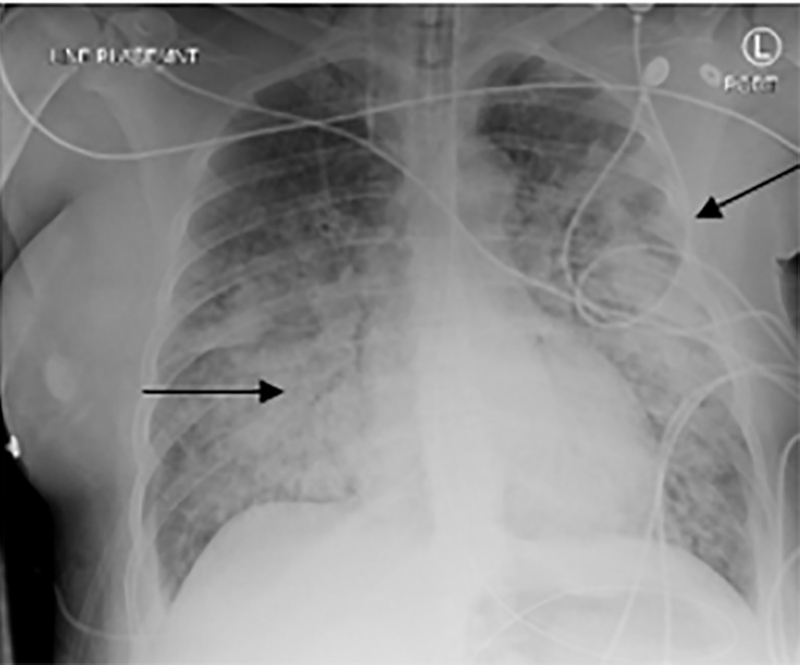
Chest radiograph of patient with arrows showing diffuse pulmonary edema
